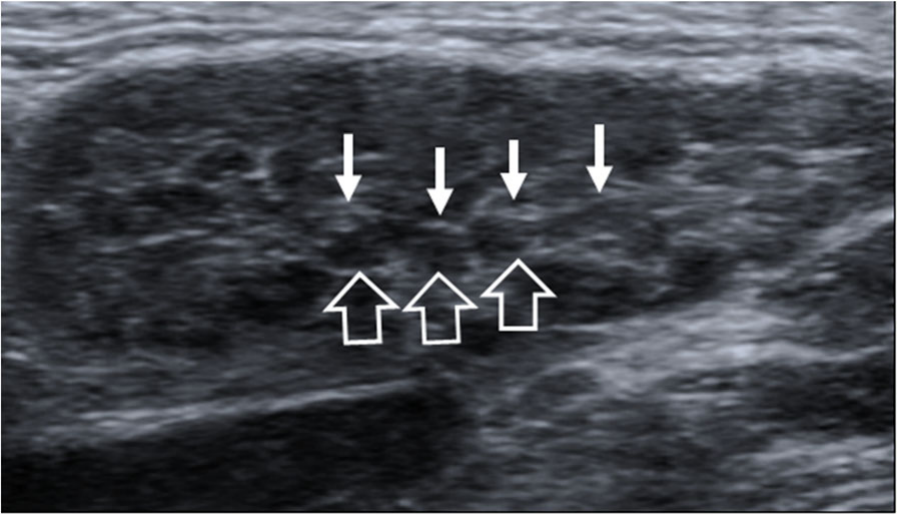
Ultrasound of a 72-year-old man with right submandibular MALToma presenting as bilateral parotid and submandibular gland swelling for 6 months. The right submandibular gland shows replacement by multiple small hypoechoic nodules of similar size (open arrows) separated by echogenic strands (arrows). The appearance overlaps with that of Sjögren’s syndrome without a dominant nodule to indicate this was MALToma
